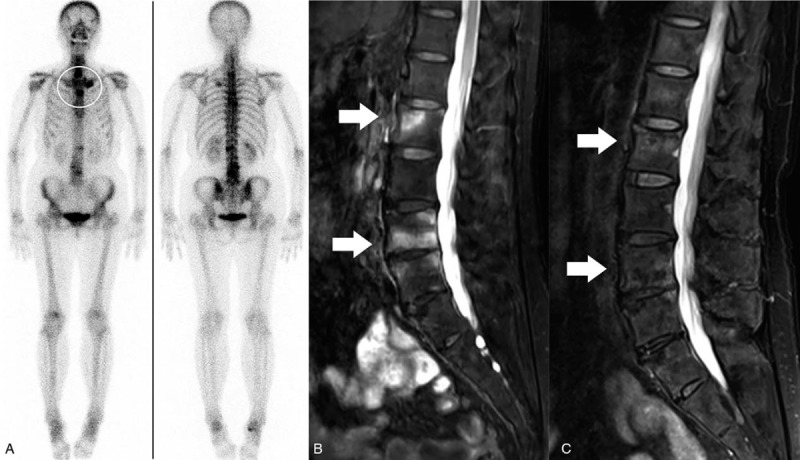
A, ^99m^Tc-MDP whole body bone scintigraphy showed the characteristic “bull's head” sign in the anterior chest wall (white circle). B, MRI (T2 fat suppression sequence) revealed the high signal intensity (white arrow), demonstrating bone marrow edema. C, Bone marrow edema disappeared (white arrow) after the treatment of Tripterygium glycosides. MRI = magnetic resonance imaging.
